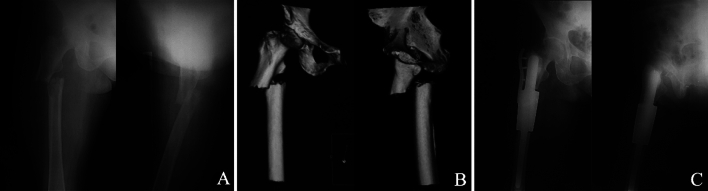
Cemented fixation of a proximal femoral prosthesis in a 53-year-old woman following resection of a metastatic breast carcinoma. (A) Anteroposterior and lateral radiographs showing a pathological diaphyseal fracture due to metastatic tumor. (B) 3D reconstruction by CT showing a pathological diaphyseal fracture, and fracture end was irregular. (C) Anteroposterior and lateral radiographs showing that screws of the auxiliary lock plate were fixed in the unilateral cortical.
